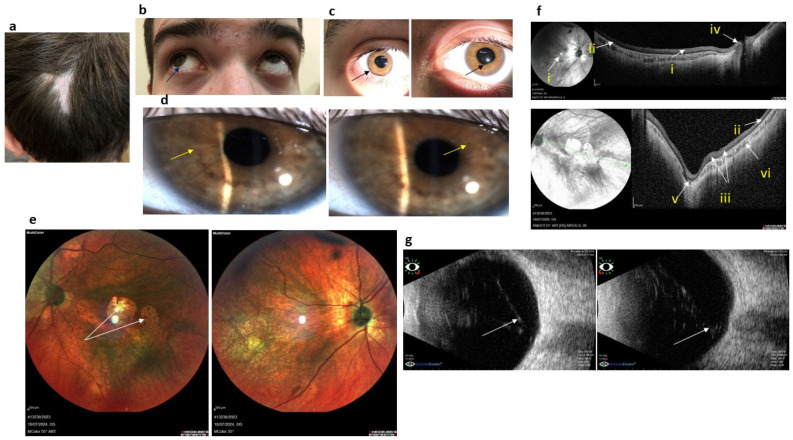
Results of examination of a boy with KS (Case 1, m 14 y.o., Family K). (a) Photo of the occipital part. The white arrow points to scarring hair loss that arose after encephalocele surgery. (b) Photo of the patient’s eyes. Arrows depict deviation of the eyeball. (c) Arrows depict iridocorneal synechia and pupil shape anomalies. (d) Slit lamp photo. Arrows depict the iris without crypts and cornea pigment precipitates. (e) Ophthalmoscopy results. Arrows point to chorioretinal dystrophic foci in the central part of the patient’s left eye. (f) OCT results. Arrows depict right foveal umbo absence (i), thinned retina (ii), undifferentiated inner retinal layers (iii), thin nerve fiber layer (iv), destructed retinal outer layer (v), and thinned choriocapillaris layer (vi). (g) Ultrasonic eye examination results. Arrows depict bilateral fiber cords in the central parts of the retina.
